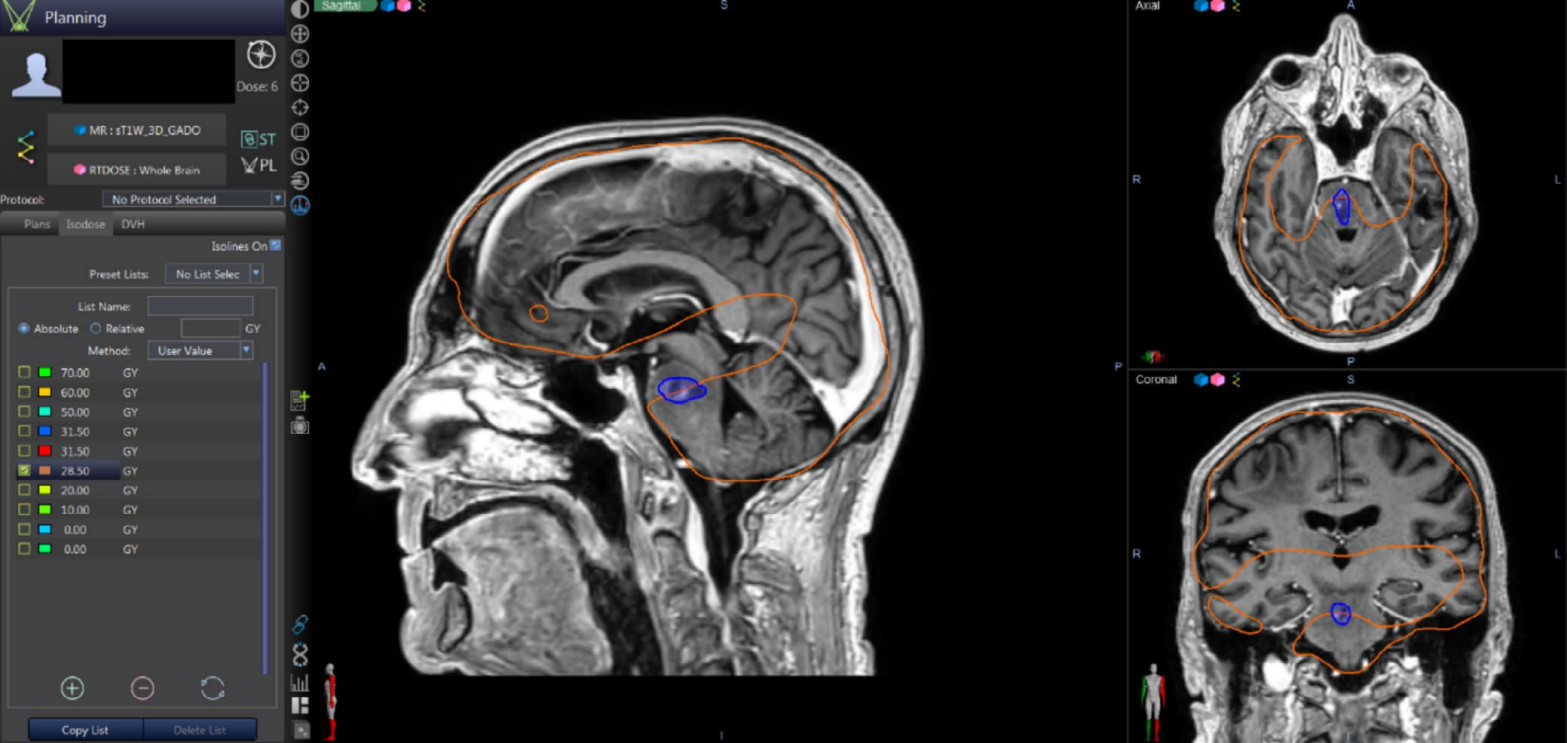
Fusion image of the intensity‐modulated radiotherapy (IMRT) plan of hippocampal avoidance whole brain radiotherapy (HA‐WBRT) showing 95% of the prescription dose (in orange) with the T1‐weighted brain MRI showing the new pontine lesion (in blue).
